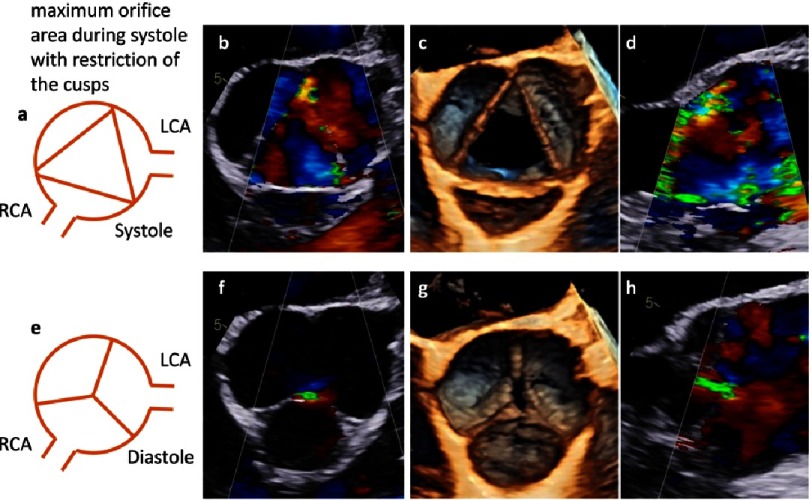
Cusp tethering and functional cusp restriction - tethering is described by reduced geometric cusp height. Scheme of the short axis view (a), color-coded 2D transesophageal short axis view (b), 3D transesophageal en-face view of the AV (c) and color-coded 2D transesophageal long axis view (d) are shown above during systole. Corresponding views are shown below during diastole (e-h).
